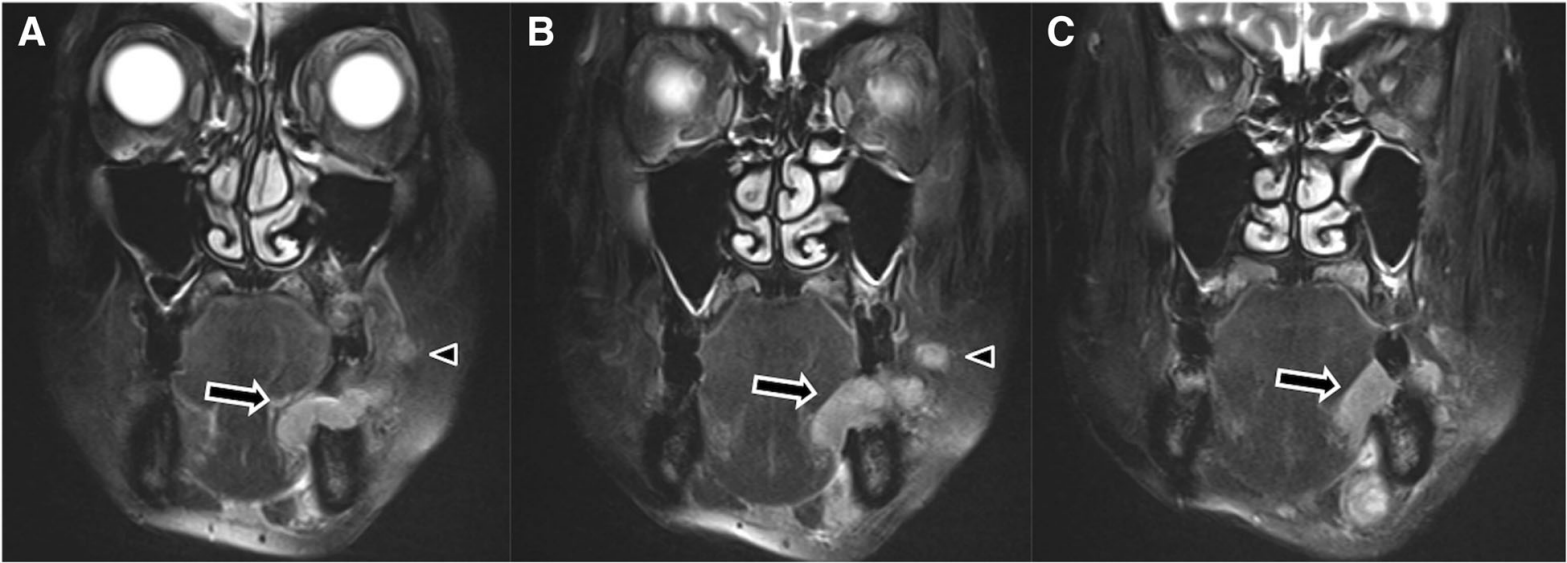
a–c Coronal fat-suppressed T2-weighted MR image showing the primary tumor (arrow) on the left side of the lower gingiva, independent of BN metastasis (arrowhead), which is embedded in the cephalad position of the primary tumor
